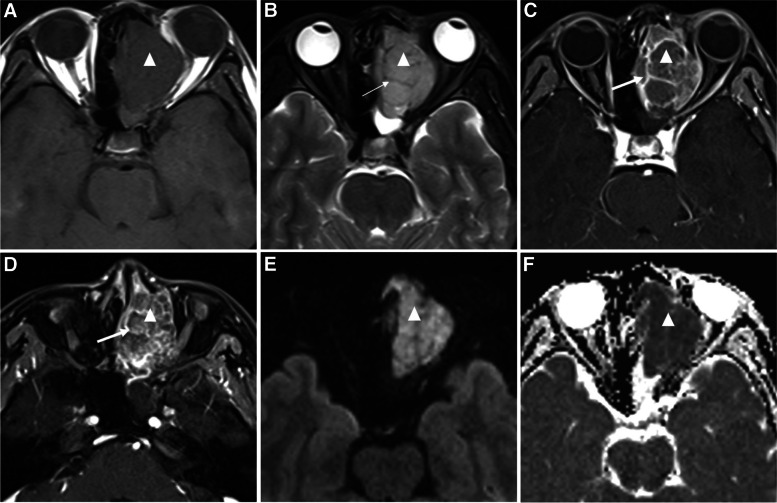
A 32-year-old female patient with ERMS. a T1-weighted, b T2-weighted, and c, d T1-weighted fat-suppressed postcontrast images show an irregular soft tissue mass (triangle) in the left ethmoid sinus along with involvement of the left orbit and sphenoid sinus. The size of the mass is 3.6 × 3.0 × 5.3 cm^3^ and the margin is ill-defined.The mass is isointense on T1WI and slightly hyperintense with low signal (thin arrow) on T2WI. After contrast enhancement, the mass is enhanced mildly and heterogeneously, and botryoid enhancement with multiple small rings (thick arrow) can be seen. e DWI shows high signal in the mass (triangle). f ADC map shows the evident low signal (triangle) with a measured ADC of 0.649 × 10^–3^ mm^2^/s
